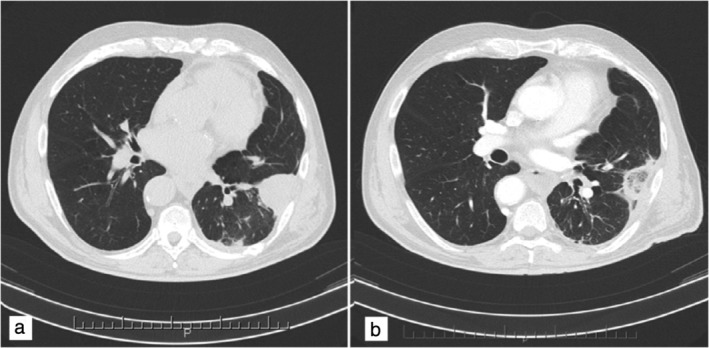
Solitary fibrous tumor of the pleura. Multi‐detector computed tomography (MDCT) revealed a focal pleural thickening at the lower left lobe (a) before and (b) three months after microwave thermal ablation. Fibrotic retraction of the lesion showed dyshomogeneous aspect due to multiple necrotic areas and cavitation within the lesion.
